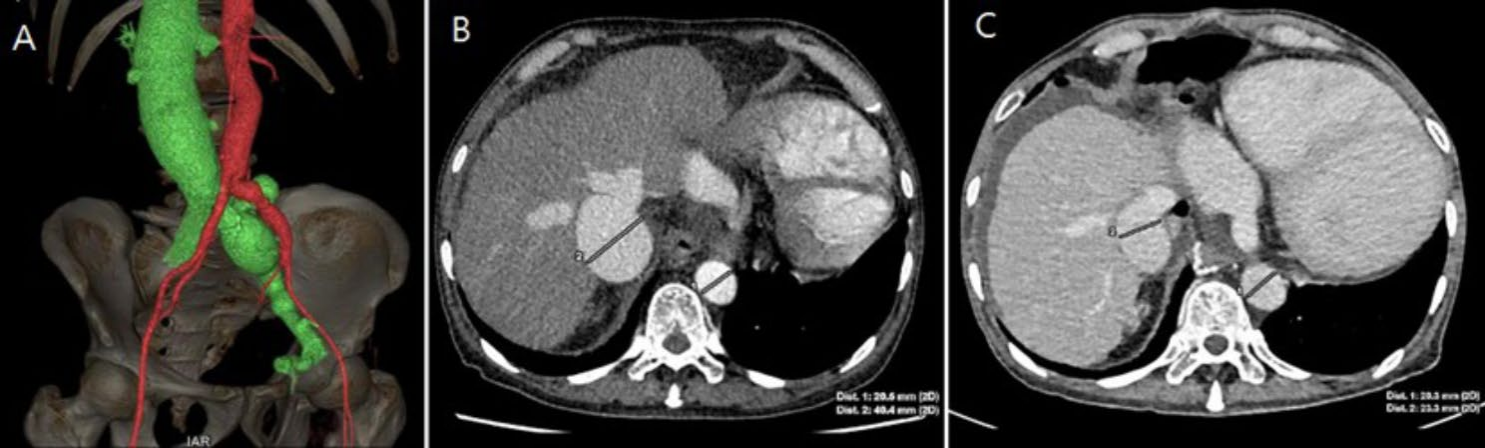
Computed tomography angiogtaphy of the inferior vena cava, left iliac vein, and right upper common iliac vein (A). Inferior vena cava diameter before the surgery (B) and after the surgery (C)
